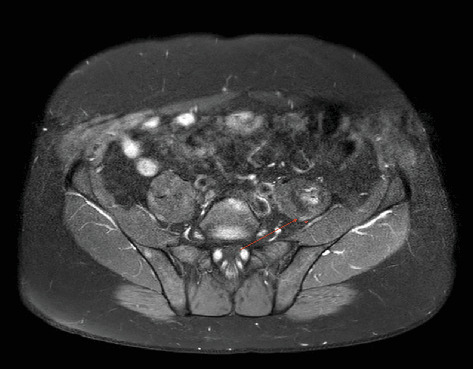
Mass originating from left psoas muscle. The red arrow showing the inferior border of the mass, asterisk showing the intersecting femoral nerve (axial T1-weighted MRI).
